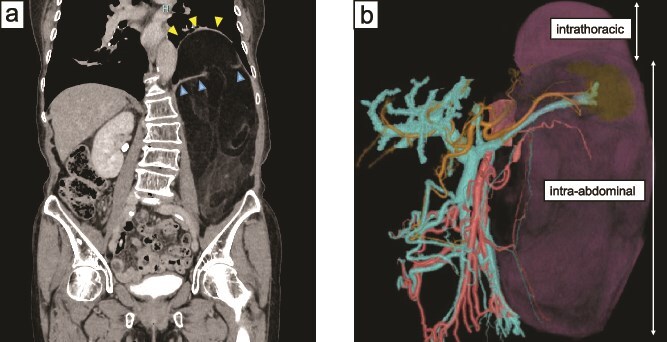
Preoperative imaging findings. (a) Contrast-enhanced CT showing a large retroperitoneal tumor protruding into the left thoracic cavity through a diaphragmatic defect. The diaphragm appears markedly thinned and stretched by the tumor (arrows). This finding suggests chronic mechanical stress on the diaphragm caused by the large tumor. (b) Three-dimensional reconstructed image demonstrating partial intrathoracic extension of the tumor through the diaphragmatic defect.
